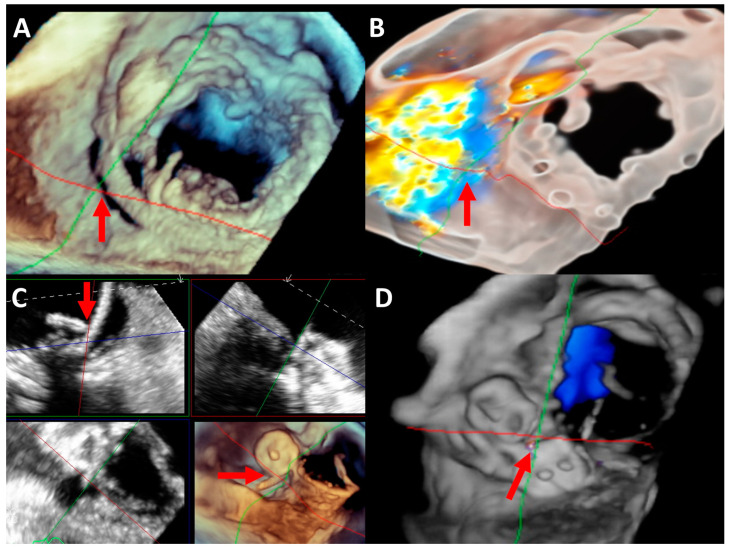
Three-dimensional transesophageal echocardiography with evaluation and guidance of paravalvular leak closure. (A,B) show preprocedural evaluation with and without color Doppler, identifying severe paravalvular leak on the posterolateral aspect of the bioprosthetic mitral valve (arrows). (C) shows during procedure after one vascular plug is deployed, the wire crossing the defect below the prior plug in anticipation for the second plug to be deployed localized with live multiplanar reconstruction imaging (arrow). (D) shows the final result after 5 vascular plugs were deployed for the paravalvular leak with color Doppler showing trivial residual regurgitation (arrow).
